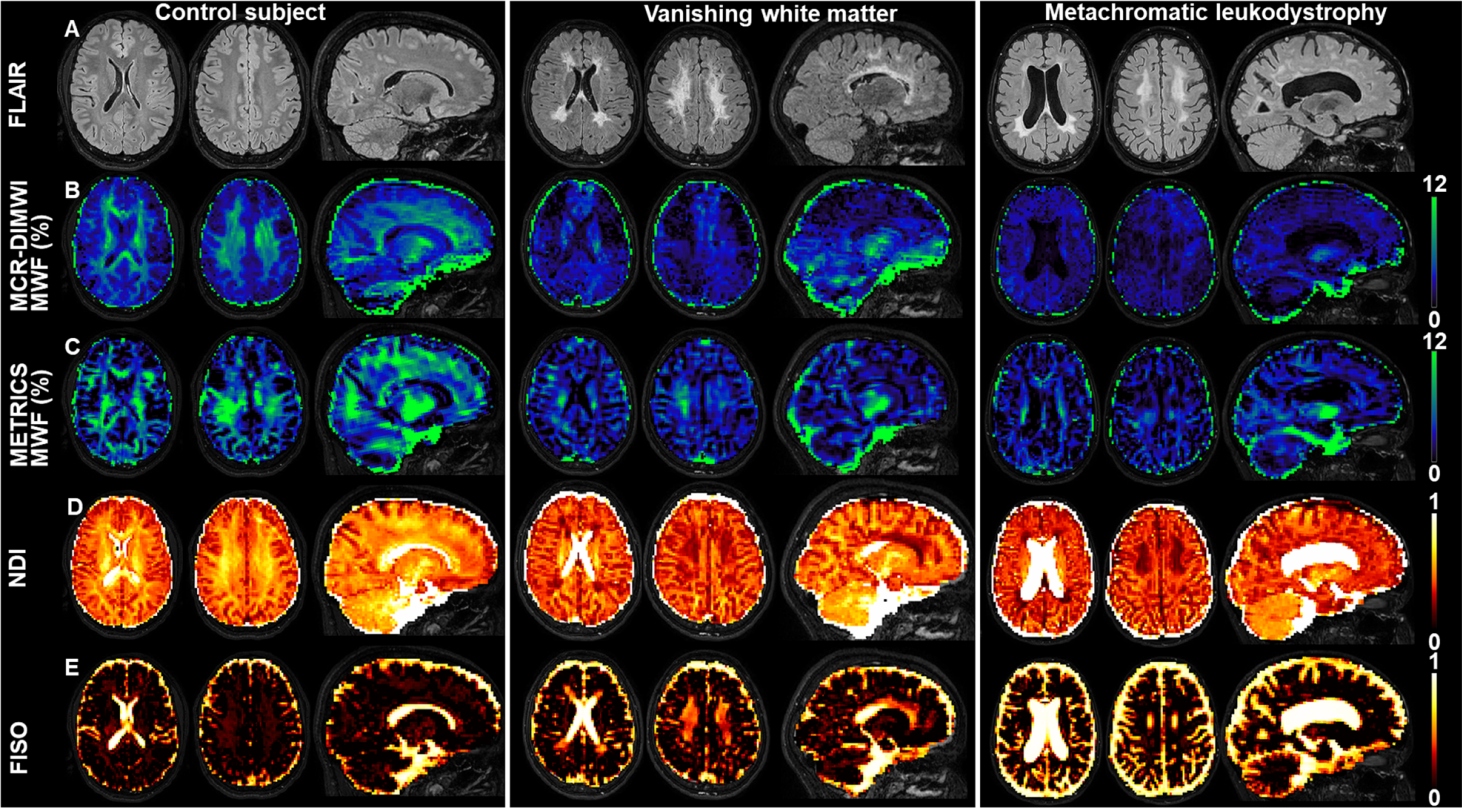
FLAIR images and quantitative MRI maps. The left columns show images of a control subject (13 years), the middle columns of a VWM patient (12 years), and the right columns of an MLD patient (15 years). Areas with FLAIR hyperintense signal intensities are seen in the patient with VWM and in the patient with MLD (A), indicating abnormal white matter. In the patient with VWM, small areas of rarefied white matter (lower FLAIR signal intensity than normal-appearing white matter) are seen in the frontal white matter. The MCR-DIMWI- and METRICS-derived MWF (respectively, B, C) are lower in the two patients than in the control subject, especially in affected white matter areas. Furthermore, NDI (D) is lower in the two patients than in the control subject, although the cerebellar white matter looks normal in the VWM subject. Despite the limited clear rarefaction and the relatively similar appearance on FLAIR of the VWM and MLD patient, FISO (E) is higher in the patient with VWM, than in the patient with MLD and the control subject. Additional maps (FA, MD, RD, T2*, T1, and IET2) are available online in Fig. S1. FLAIR, fluid-attenuated inversion recovery; VWM, vanishing white matter; MLD, metachromatic leukodystrophy; MWF, myelin-water fraction; MCR-DIMWI, multi-compartment relaxometry diffusion-informed myelin water imaging; METRICS, multi-echo T2 relaxation imaging with compressed sensing; FISO, free (isotropic) water fraction; NDI, neurite density index
